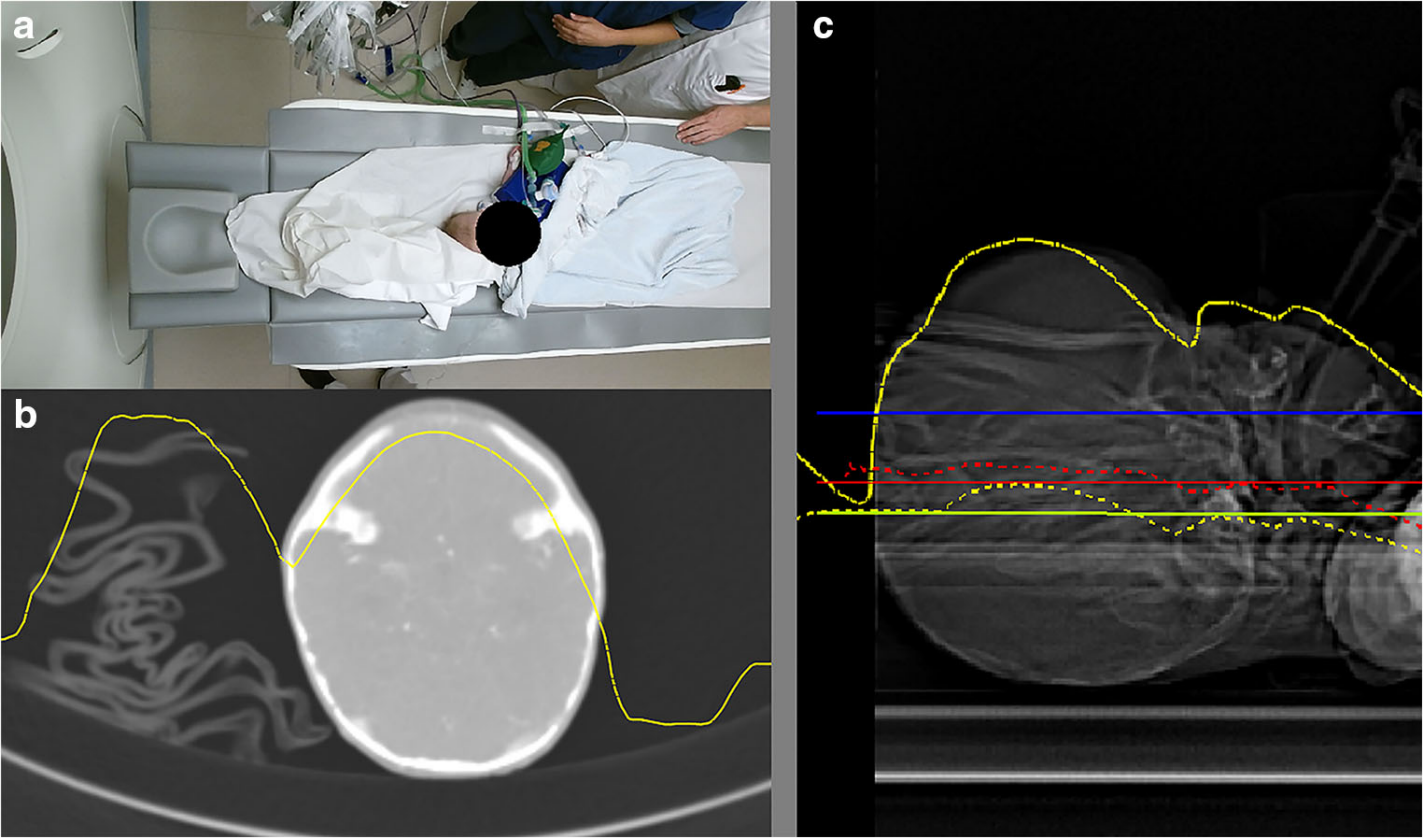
Case presentation of a 3-month-old child wrapped in a blanket with a breathing support and wires where accurate positioning is challenging for both the 3D camera and the radiographers. a Color image taken by the 3D camera system. b Axial image of the brain with depth measurements (yellow line) by the 3D camera. c Sagittal image of the head with patient positioning accuracy: blue horizontal line: patient isocenter estimated by the radiographer, green horizontal line: algorithm isocenter estimated by the camera, green dotted line: algorithm isocenter curve, red horizontal line: average patient isocenter (ideal table height), red dotted line: patient isocenter per axial cross-section, yellow line: depth measurements
